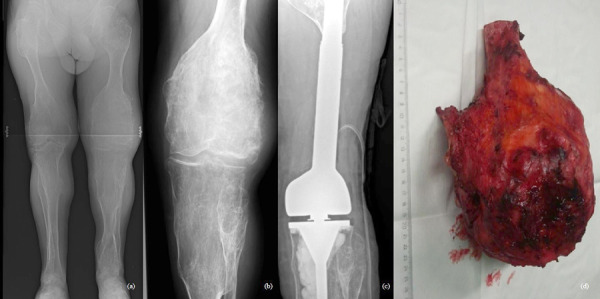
a-b) On December, 2015, radiographs showed multiple destructive lesions in the legs; c) on March, 2016, resection of the left bottom part of femur and arthroplasty with megaprosthesis; d) resected tumor – distal part of femur. Chondrosarcoma G3
